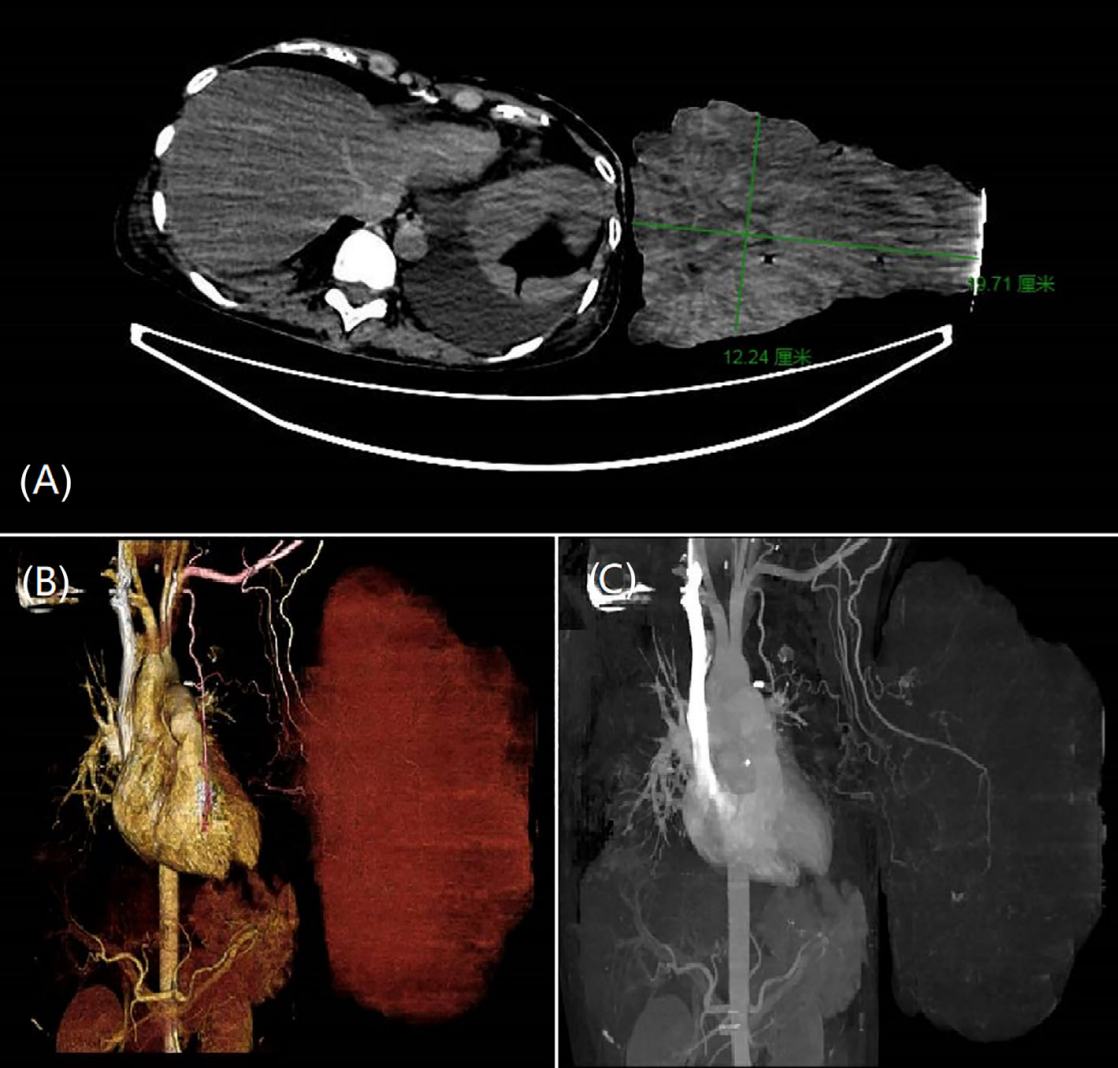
Initial computed tomography (CT) scan showing a heterogenous mass, sagittal plane (Figure 3A), transverse plane (Figure 3C), CT angiography (Figure 3B).
